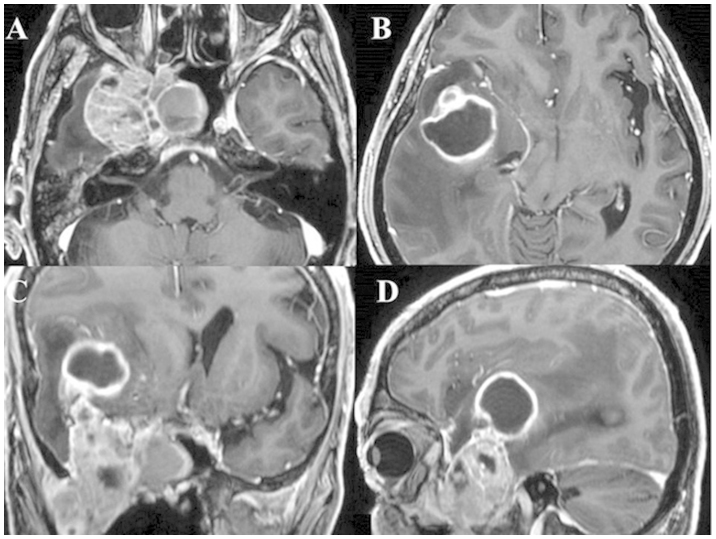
Enhanced magnetic resonance imaging performed on admission. (A and B) Axial, (C) coronal and (D) sagittal views showing a solid mass extending from the maxillary sinus to the middle cranial base.
